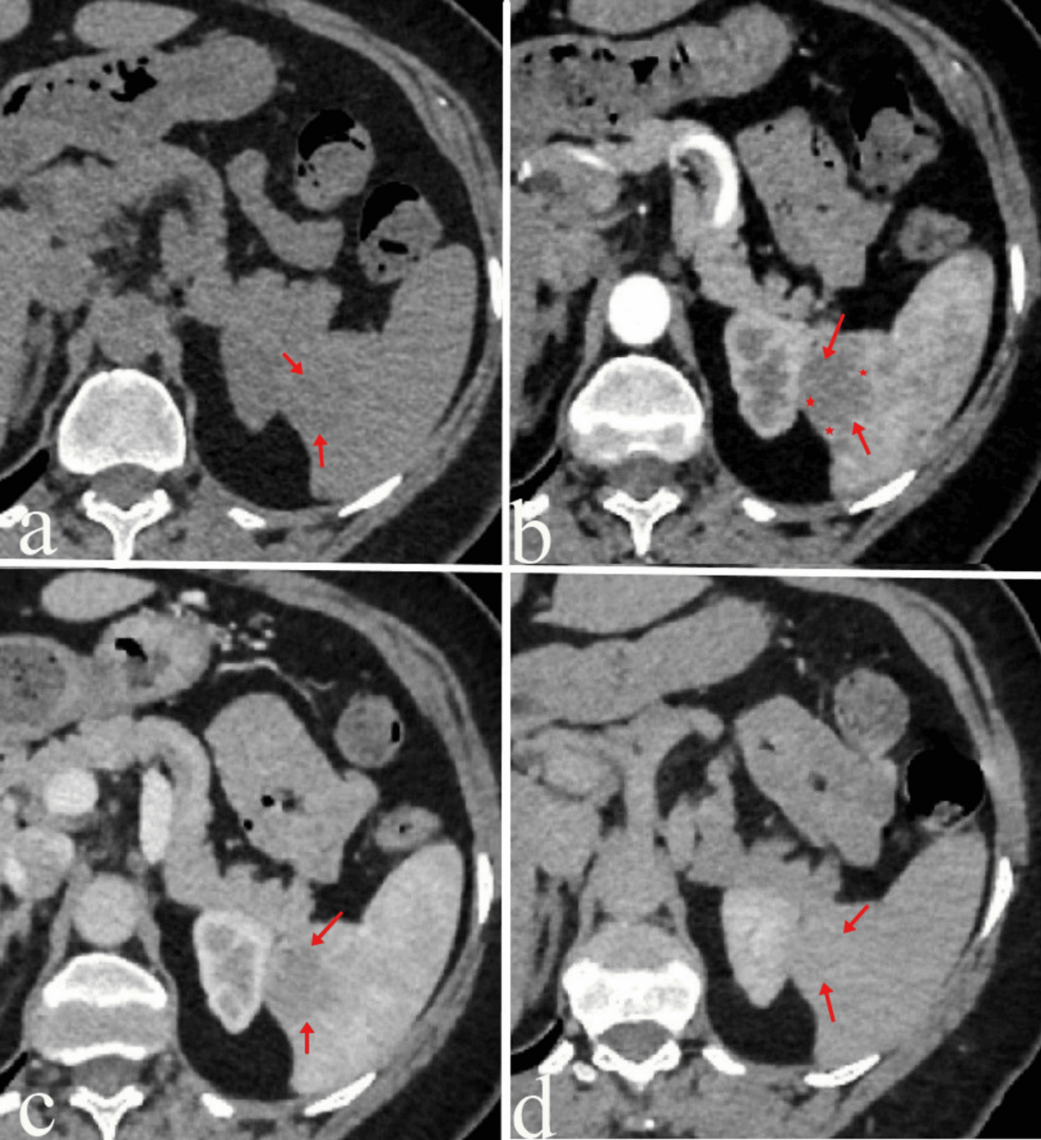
Dynamic contrast-enhanced axial CT examination On non-contrast axial CT imaging (a), a hypodense mass lesion measuring 26x20 mm with mildly lobulated contours is observed in the inferior spleen (red arrows). On post-contrast images (red arrows), the lesion demonstrates peripheral nodular enhancement during the arterial phase (b) (red asterisks) and progressive centripetal enhancement during the portal phase (c). In the late venous phase images (d), the lesion shows enhancement similar to the surrounding splenic parenchyma.
